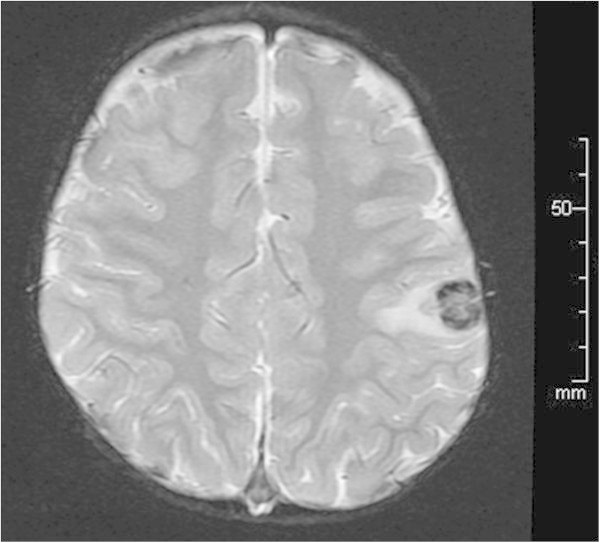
T2-weighted magnetic resonance imaging revealed an intracranial tumor in the left parietal lobe, accompanied by extensive peritumoral edema.
